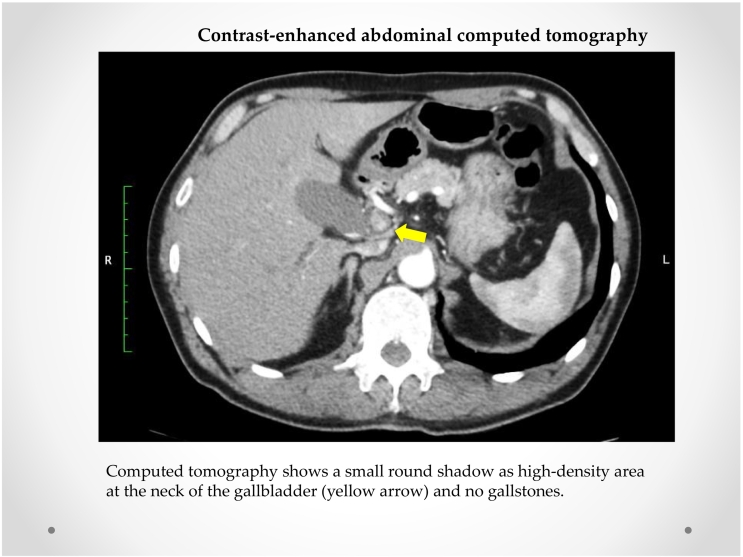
Contrast-enhanced abdominal computed tomography (CT) CT shows a small round shadow at high-density areas at the neck of the gallbladder (white arrow) and no gallstones.
